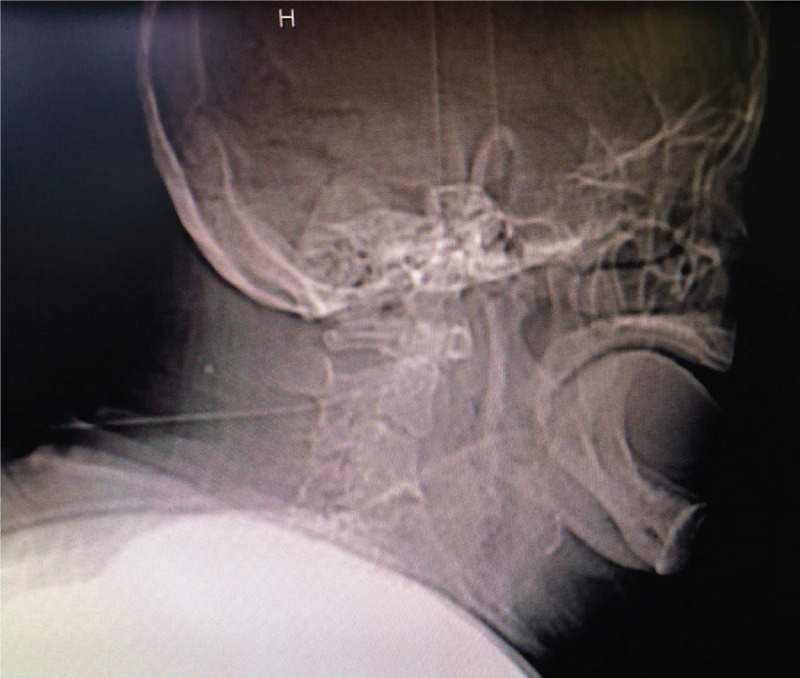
The CT image of patient's head and neck.
